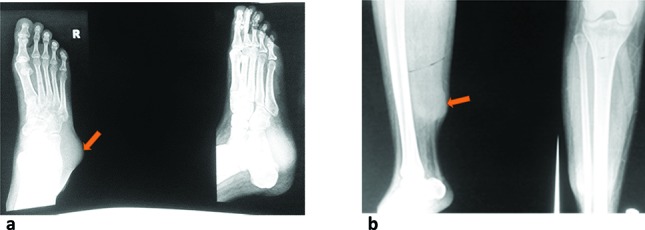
Plain radiographs showing homogenous soft tissue masses over the right ankle (a) and calf (b).
